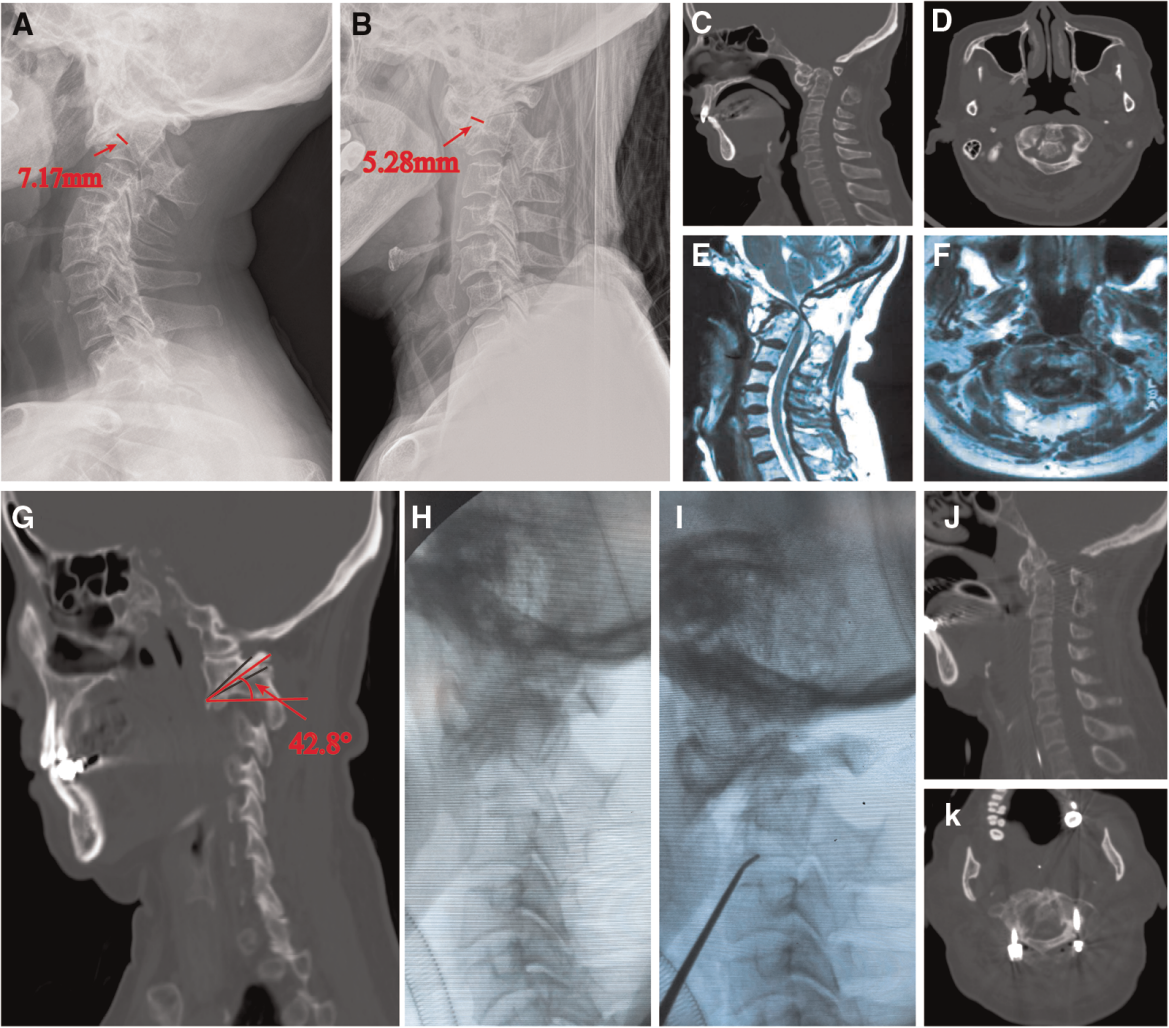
(A,B) A 71 years old female with AAD, the x-ray showed the results of traction: ADI before and after traction is 7.17 mm and 5.28 mm, RI is 26.36%. (C,D) Computed tomography (CT) was taken to show the condition of dislocation and fusion. (E,F) Magnetic resonance imaging (MRI) showed the compression of cervical spine. (G) We measured the SAAJI in the sagittal CT, and the result is 42.8°. (H–K) Anterior release and posterior operation was conducted for her, which get a satisfied reduction.
